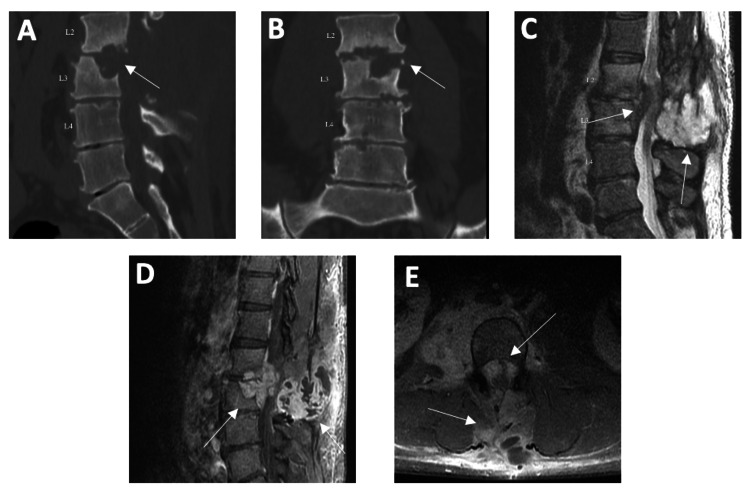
CT (A and B) and MRI (C, D, and E) of the lumbar spine taken during first rehospitalization. CT scans include sagittal T1 without contrast (top left) and coronal T1 without contrast (top middle). CT in both sagittal (A) and coronal (B) views demonstrates increased sclerosis of the endplates at the L2-3 level with endplate erosion and vertebral body destruction prominent along the inferior aspect of the L2 vertebral body consistent with a discitis and acute infection. MRI shows an epidural soft tissue lesion seen at the level of L2-3 and seen clearly on sagittal T2 (C), which also enhances on T1 post contrast (D and E) and enhances and compresses the canal and nerve roots. Also appreciated is a 5.6 × 3.8 soft tissue enhancing mass present posteriorly at this level slightly inferior to the epidural abscess, which contains multiple internal septations (E).
